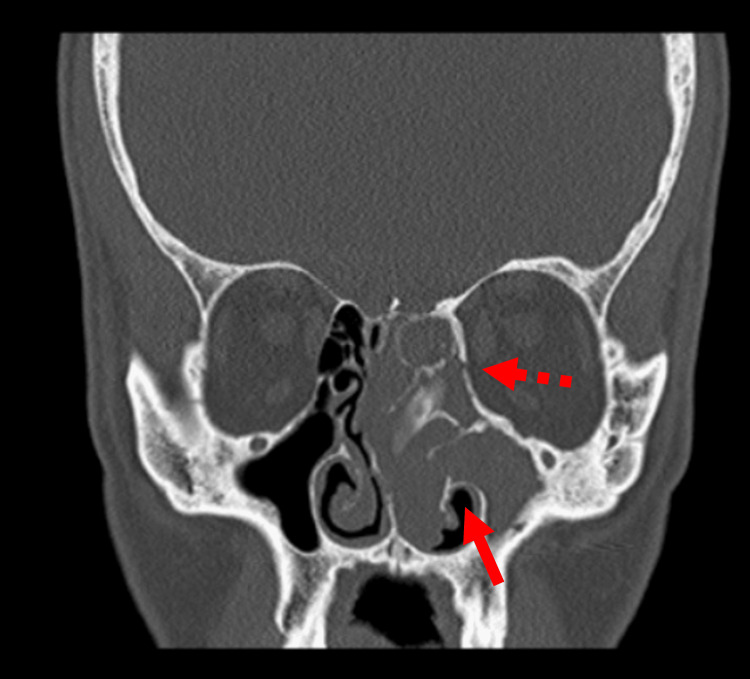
CT scan (bony window) coronal cut shows soft tissue lesion filling the left maxillary sinus extending into the nasal cavity with widening of the infundibulum. Focal hyperostosis at the anterior ethmoid air cells. The left ethmoid and left frontal sinuses also appeared filled with the mass. There was suspicious bony erosion, especially near the anterior medial orbital wall. Soft tissue lesion filling left maxillary sinus and nasal cavity (red arrow). Bony erosion near anterior medial orbital wall (red dashed arrow).
